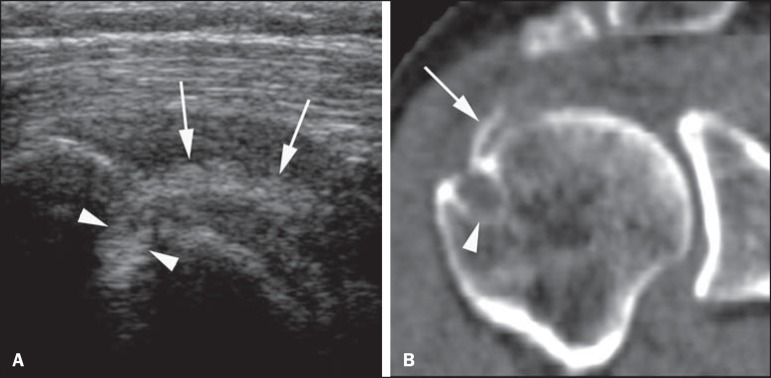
Male, 56-year-old patient. A: Sonographic image in the long
